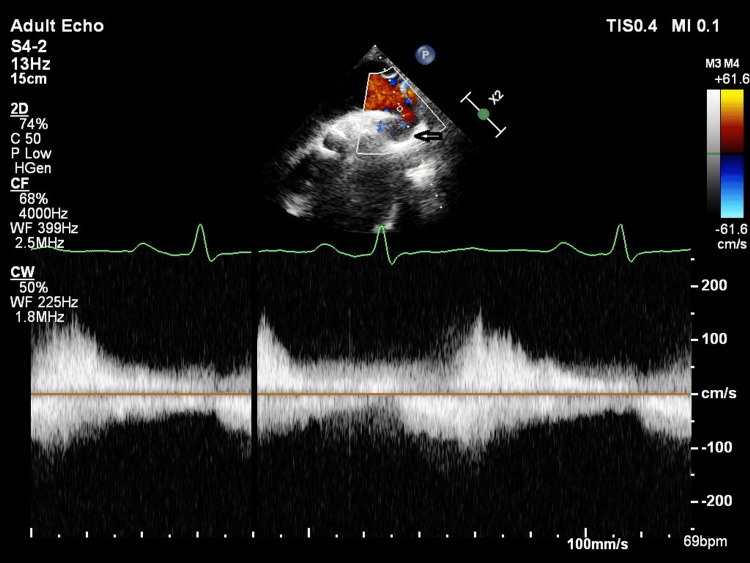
Transthoracic echocardiography Suprasternal view showing tapering of the descending thoracic aorta (arrow) and Doppler interrogation revealing no significant pressure gradient across the narrowing.
